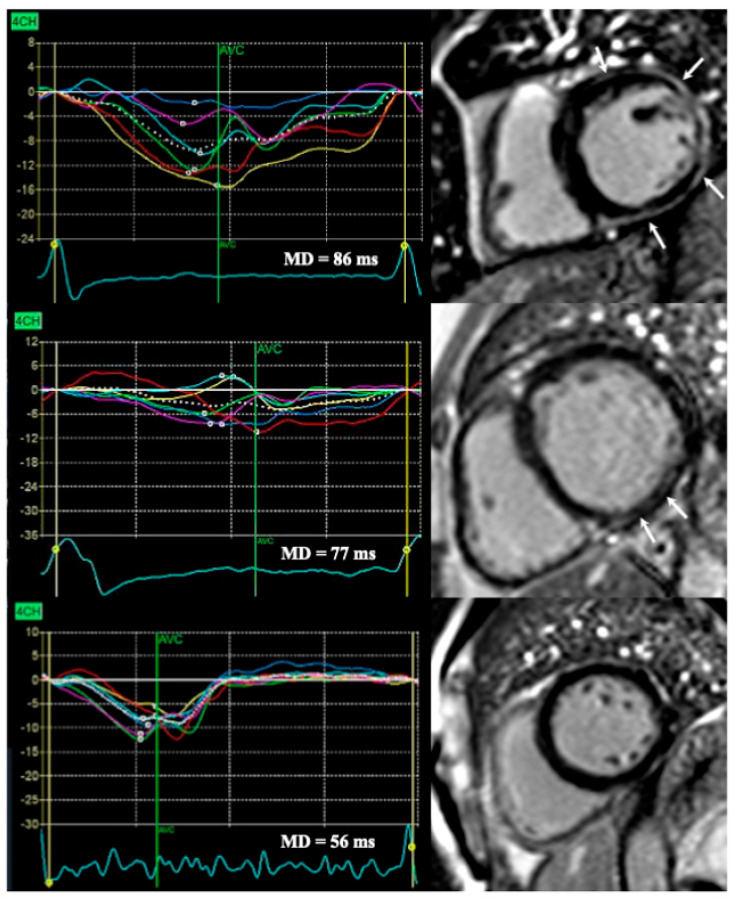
Relationship between MD by echocardiography and focal MF by cardiac magnetic resonance in representative DCM patients. The first case shows a patient with extensive MF (white arrows), the second one with limited MF (white arrows), and the third one without MF, each presented together with corresponding MD.
